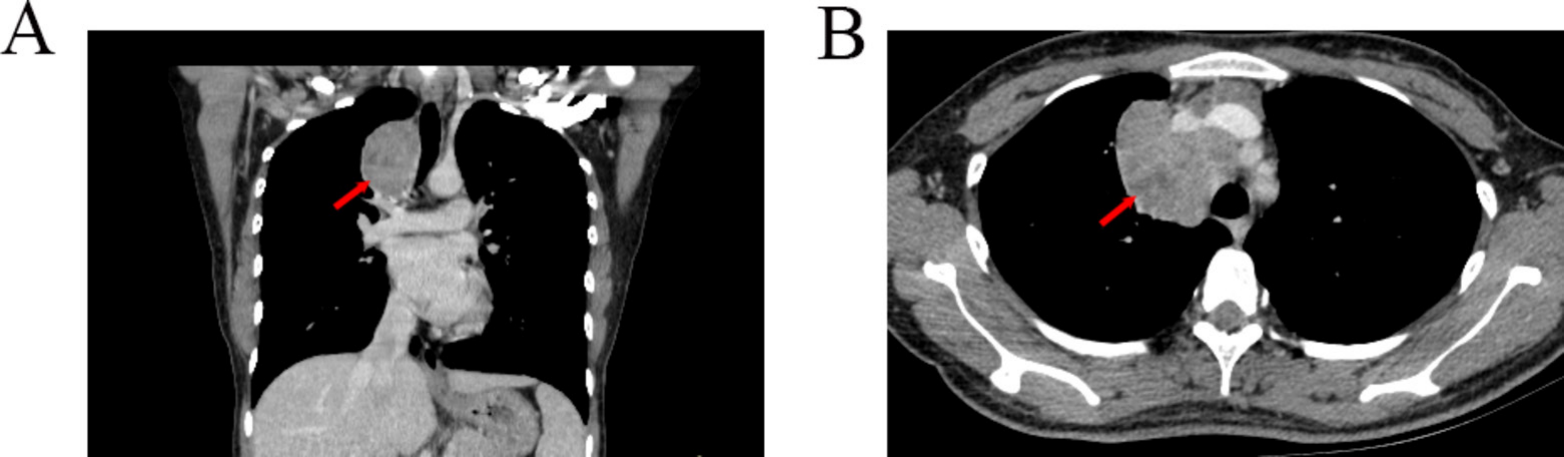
Computed tomography scan revealing a mediastinal mass that compresses the surrounding tissue.
